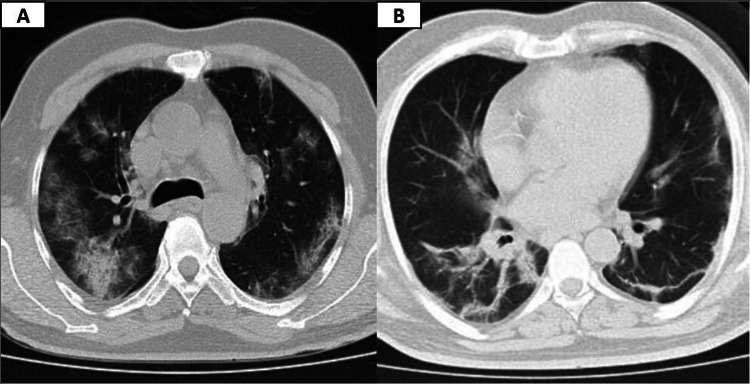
(A) High resolution computed tomography chest showing ground-glass opacity with inter and intralobular septal thickenings and (B) high resolution computed tomography showing subpleural bands and vascular dilatation
